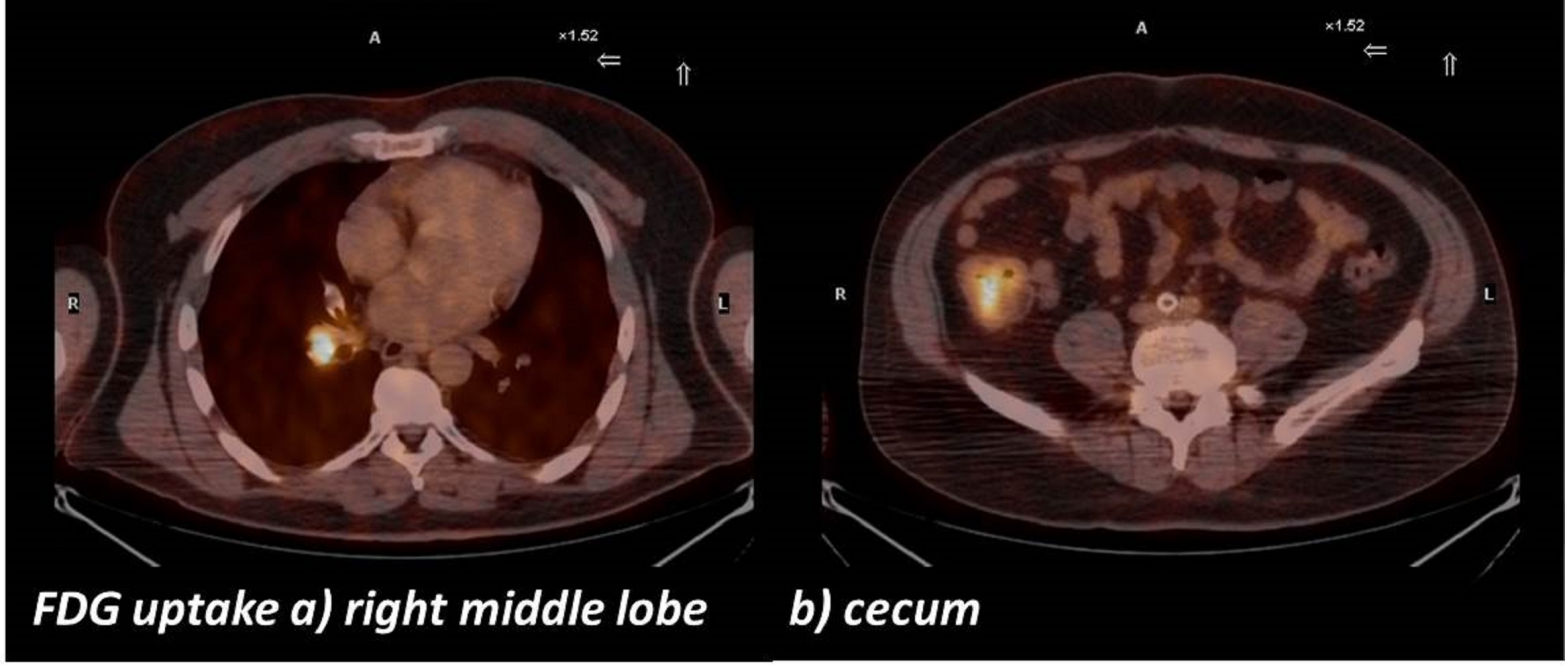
PET Scan Images FDG-uptake is shown in the a) right middle lobe, and b) the cecum.
